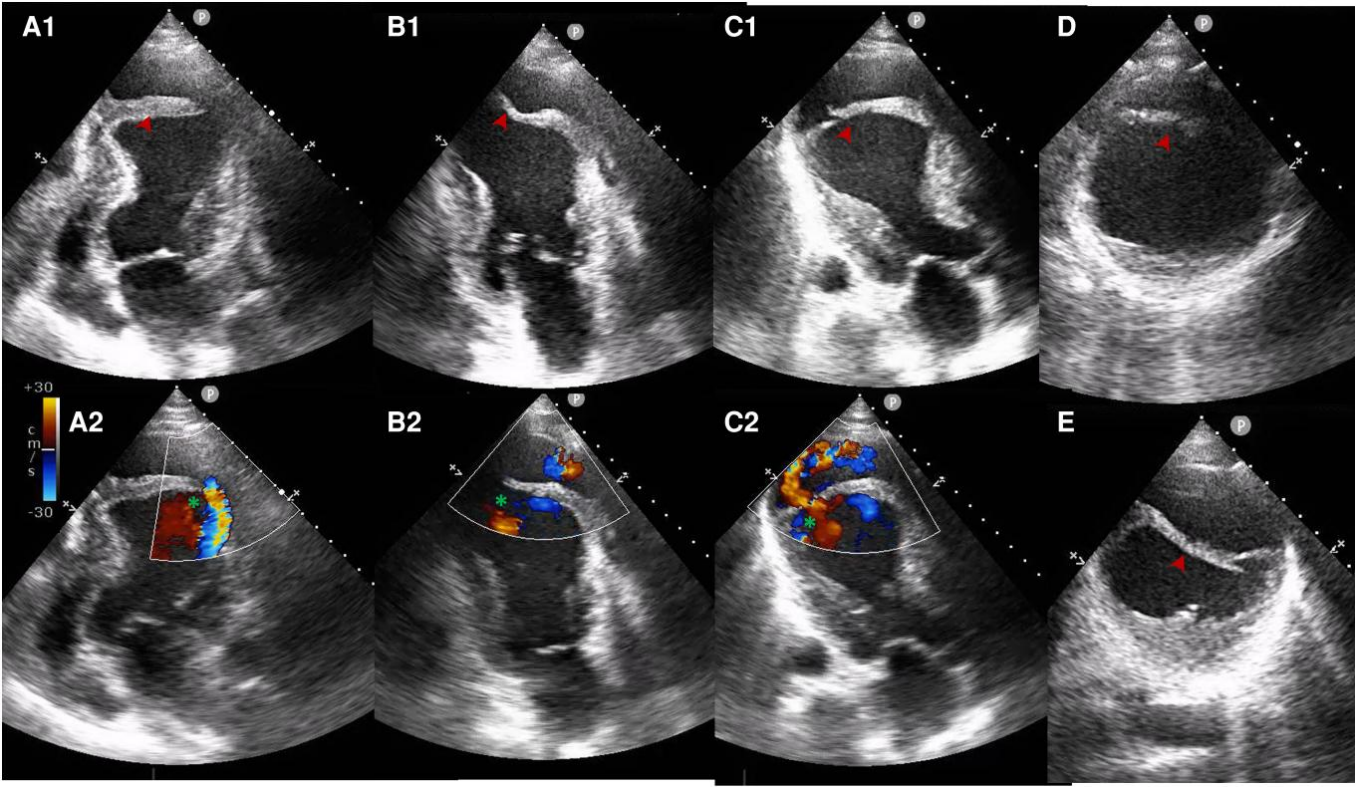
TTE in Patient 4. A4C (*A1*), A2C (*B1*), and apical three-chamber (A3C) views (*C1*) show a very large apical, anterior, and anteroseptal ID. We note a ‘flap-like’ structure separating the neo-cavity from the LV with a clear solution of continuity (arrowheads). Doppler images in the aforementioned views (*A2*, *B2*, and *C2*, respectively) show bidirectional flow (asterisk) between the LV and the IMDH. This continuous high pressure blood flow is with high level of suspicion the cause for the rapid and outstanding growth of the false-pseudoaneurysm.
